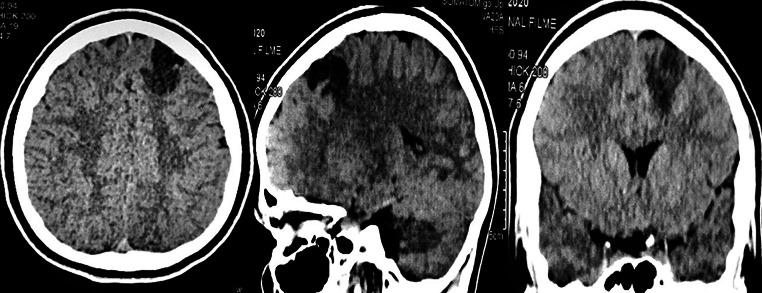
Axial, coronal, and sagittal noncontrast CT images demonstrate a well-defined, hypodense lesion in the left frontal lobe. The lesion is situated between the superior and middle frontal gyri, is in contact with the dura mater, and indents the cortical surface without mass effect.
